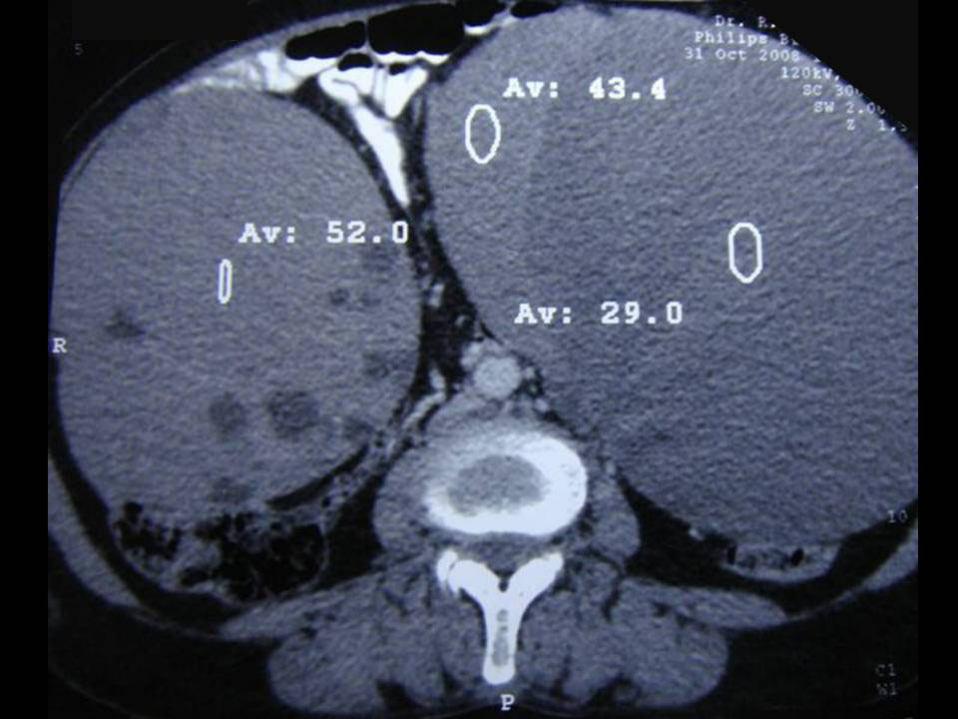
CT scan of abdomen showing two large cystic masses arising from pelvis.
